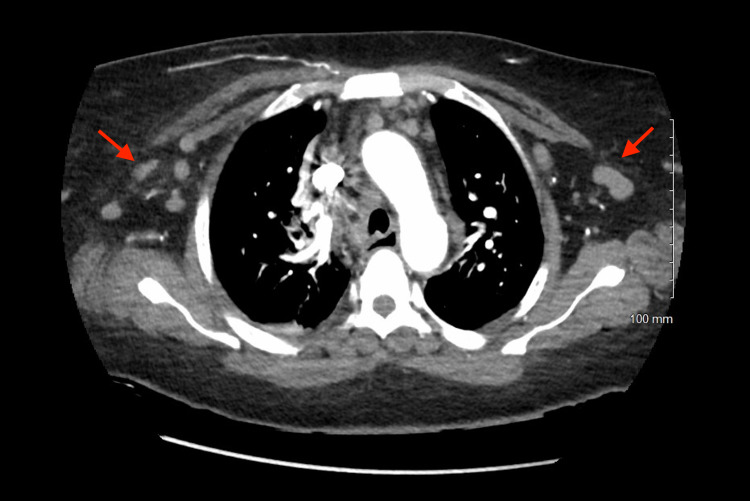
CT pulmonary angiography showing bilateral axillary lymphadenopathy (red arrows).
